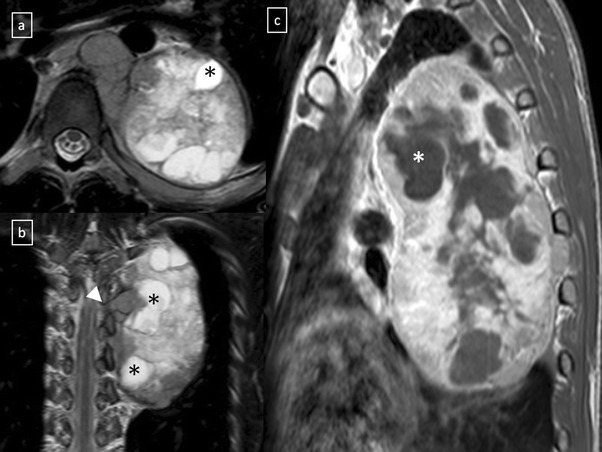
Neuroblastoma in a 20-year-old man. Axial (a) and coronal (b) T2-weighted MR images, and sagittal (c) contrast-enhanced T1-weighted MR image demonstrate an expansive and heterogeneous mass in the left paravertebral space which shows cystic degeneration within (*) as well as an intensive enhancement (in c). Note the spinal involvement (*arrowhead* in b)
